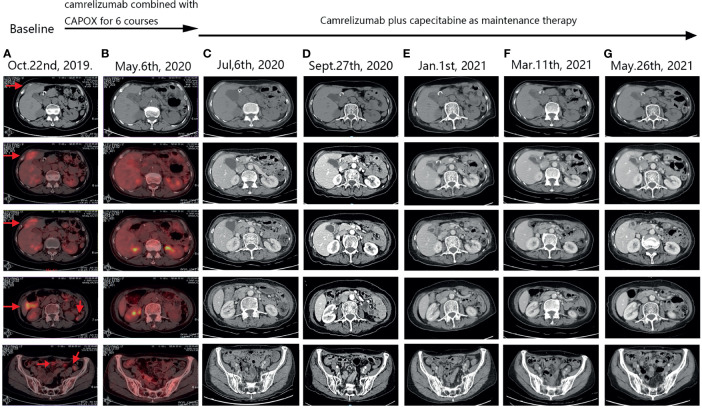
PET-CT and follow-up CT images. Arrows indicate tumors. (A) After the patient’s tumor recurred, pre-treatment positron emission tomography (PET)-computed tomography (CT) scan suggested metastatic lesions in the right lower chest wall, invasion of the adjacent peritoneum, and hepatic S4 involvement. Multiple patchy and nodular metastases in peritoneum of abdomen and pelvis. (B) After camrelizumab combined with CAPOX for 6 courses, positron emission tomography (PET)-computed tomography (CT) scan showed that the original metastatic lesions on the right lower chest wall disappeared, and the original multiple metastatic lesions on the peritoneum disappeared. No new tumors were observed in other organs. (C–G) During the period of camrelizumab plus capecitabine as maintenance therapy, regular CT scans suggested that the condition was stable, the original tumor lesions disappeared, and no new tumor lesions were found.
